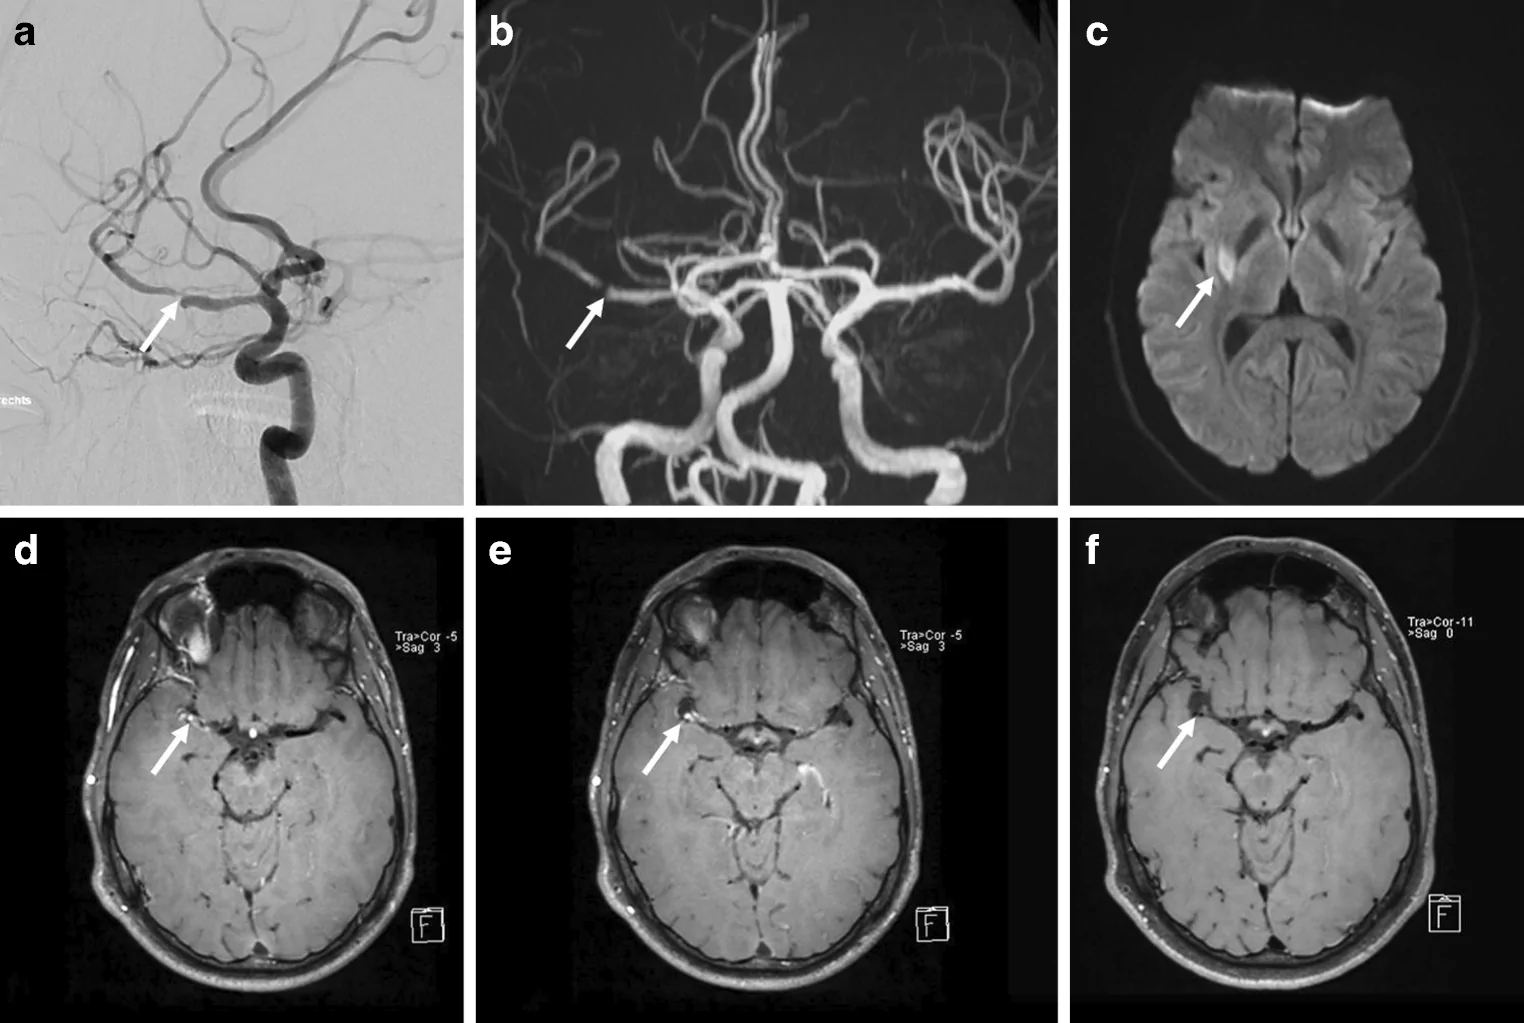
Primary CNS vasculitis in a 23 year old man suffering from acute left-sided hemiparesis; cerebrospinal fluid (CSF) analysis: no pleocytosis, slight elevated protein content. a–c digital subtraction angiography (DSA) and MR angiography showing severe stenosis in the distal M1-segment right (a, b arrow); lacunar infarct (c arrow) on diffusion weighted imaging (DWI; b = 1000 s/mm^2^) (c arrow;); d–f post contrast T1 WI (black blood sequence) disclosing circumscribed vessel wall enhancement of the right-sided distal M1- and proximal M2-segment (d, e arrow). Complete resolution of imaging findings is depicted on a follow-up study at ten months following immunomodulatory therapy (f arrow)
